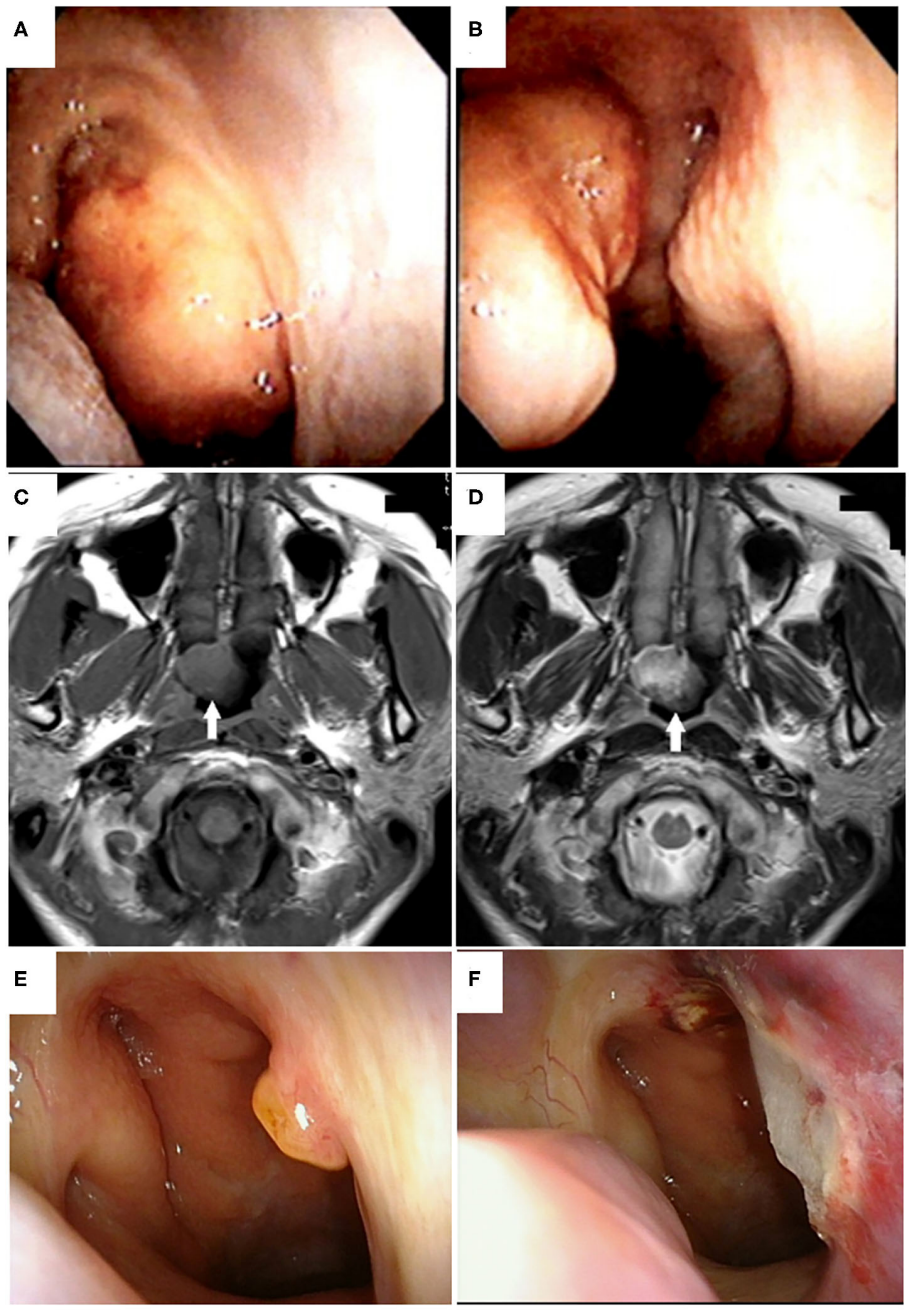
Tumor appearance on nasopharyngeal endoscopy and magnetic resonance imaging (MRI). Endoscopic findings of the nasopharyngeal tumor viewed from the right (A) and left (B) nasal cavities at the first visit to the previous hospital are shown. A pedunculated polypoid tumor originating from the posterior edge of the nasal septum was found in the epipharynx. Horizontal views of plain T1-weighted (C) and T2-weighted magnetic resonance imaging (D) of the head showed a tumor of ~20 mm in diameter located in the epipharynx originating from the posterior edge of the nasal septum without invasive or destructive findings (white arrows). T1- and T2-weighted images showed the same or slightly higher intensities compared to that of the nasal concha. Preoperative (E) and postoperative (F) endoscopic findings of the nasopharyngeal tumor viewed from the right nasal cavity in our hospital. In the preoperative view, the main portion of tumor had disappeared and only the pedunculated portion remained (E). The tumor was endoscopically resected with a 5-mm safety margin (F).
